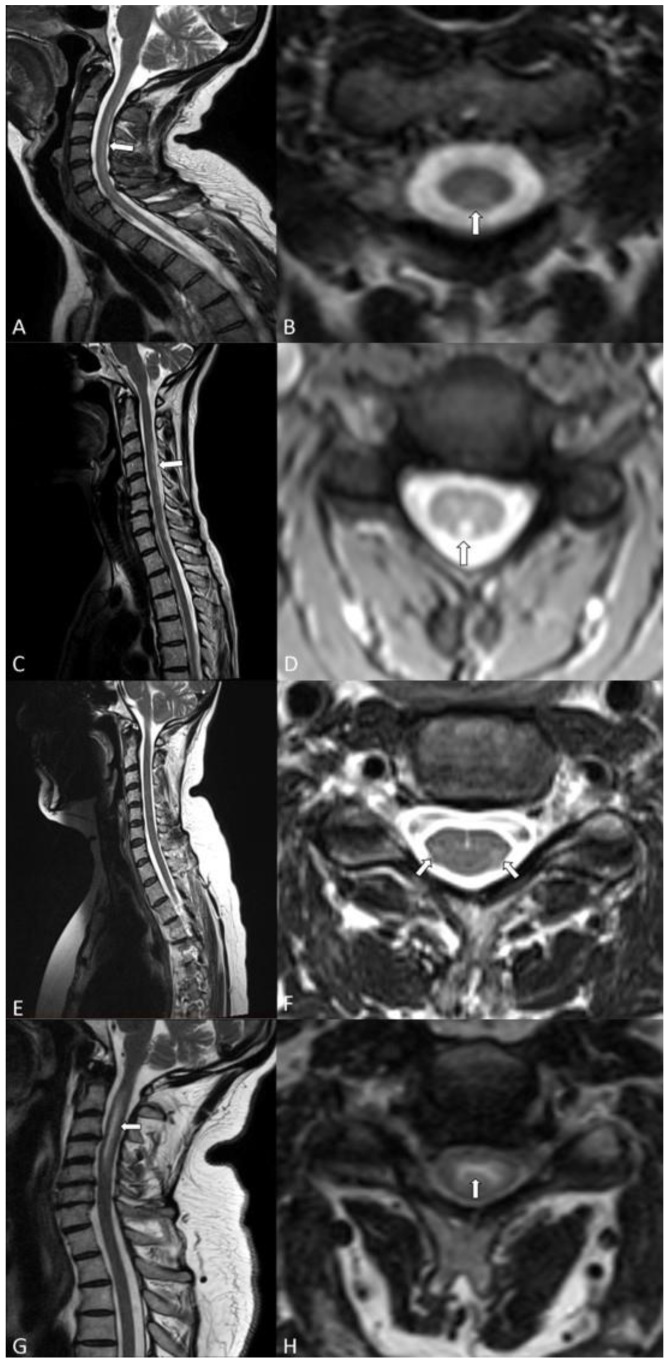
Cervical spinal cord MRI of all patients, with sagittal (left) and axial (right) T2-weighted sequences. (A,B) *Patient 1:* White arrow indicates a right-sided homogeneous T2-hyperintense lesion in the posterior columns. (C,D) *Patient 2:* White arrow indicates a T2-hyperintense longitudinally extensive spinal cord lesion in the posterior columns. (E,F) *Patient 3:* White arrows indicate a long segment T2 lesion of the lateral pyramidal tracts and lateral spinothalamic tracts. (G,H). *Patient 4:* White arrow indicates a long segment T2-hyperintense lesion mainly involving the central grey matter.
